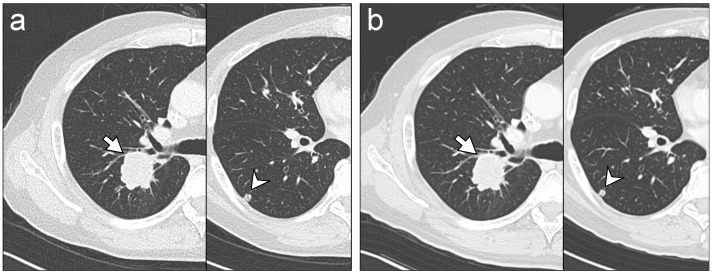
Representative chest CT images. Images were acquired on separate occasions from a 66-year-old male who received chemotherapy for lung metastases after colon cancer surgery. The readers identified lung metastasis lesions (arrows and arrowheads) on tin-filtered 100 kV (TF100kV) images (a) and standard 120 kV (ST120kV) images (b) with equal reliability, regardless.
